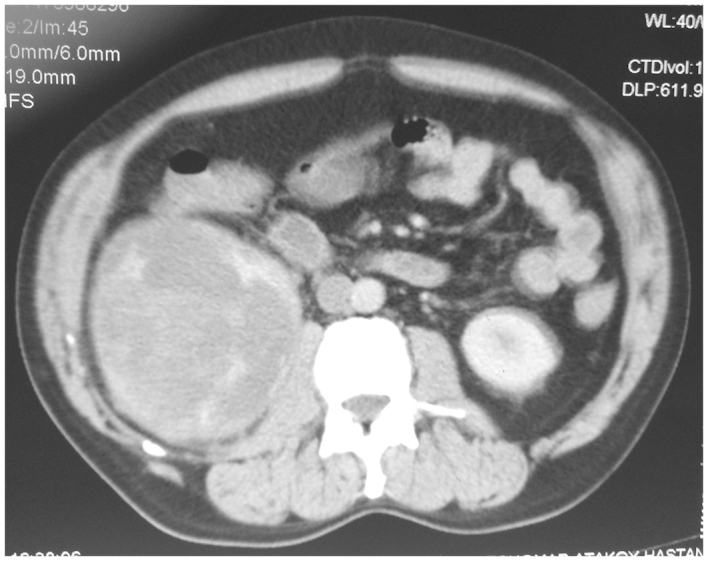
Contrast-enhanced computed tomography revealing a centrally necrotized and hemorrhagic right-sided renal mass with heterogenous peripheral enhancement.
